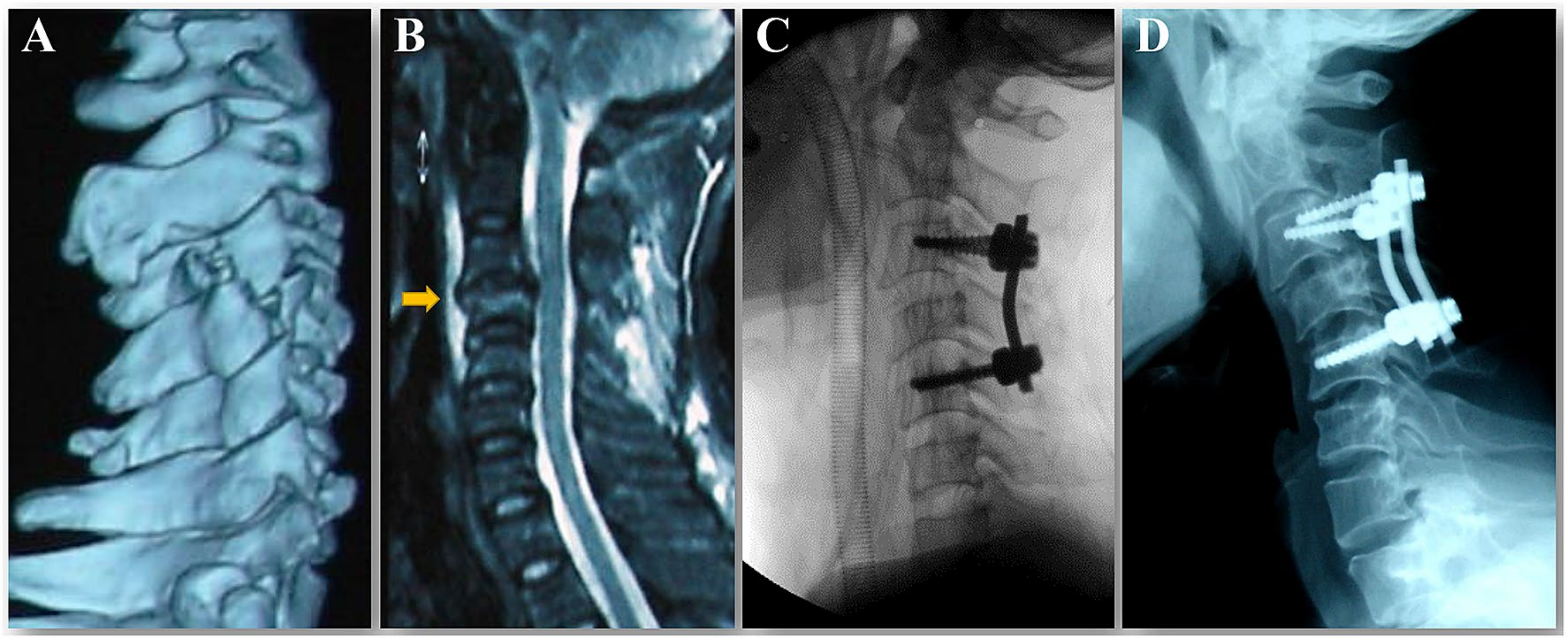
A 39-year-old man who was diagnosed as type IIIA injury pattern of UCLF. Preoperative CT three-dimensional reconstruction showed that a vertical fracture of the C4 right lateral mass created anterior–posterior separation with invagination of the inferior articular process of the cephalic adjacent vertebra (A). Cervical vertebral body compression fracture, disc and anterior longitudinal ligament injury, and hematoma in the prevertebral space (yellow arrow) were discovered on sagittal T2-weighted MR image (B). The patient underwent posterior facet release and reduction, and C3-5 pedicle screw fixation (C). A mild late kyphosis deformity because of intervertebral space subsidence and vertebral collapse was found at 8 months follow-up after surgery (D).
